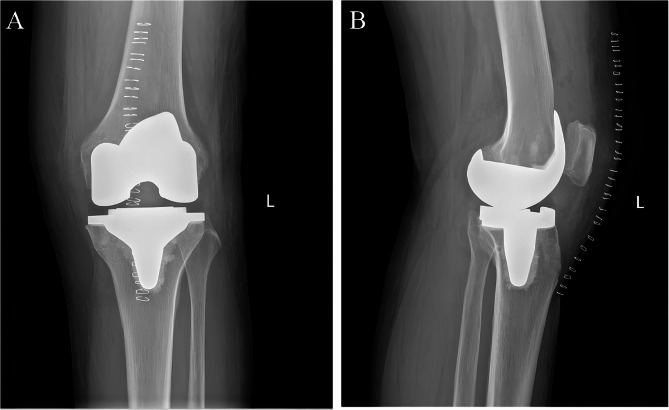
Post operation X-ray (AP and lateral view) of left knee after total knee arthroplasty
